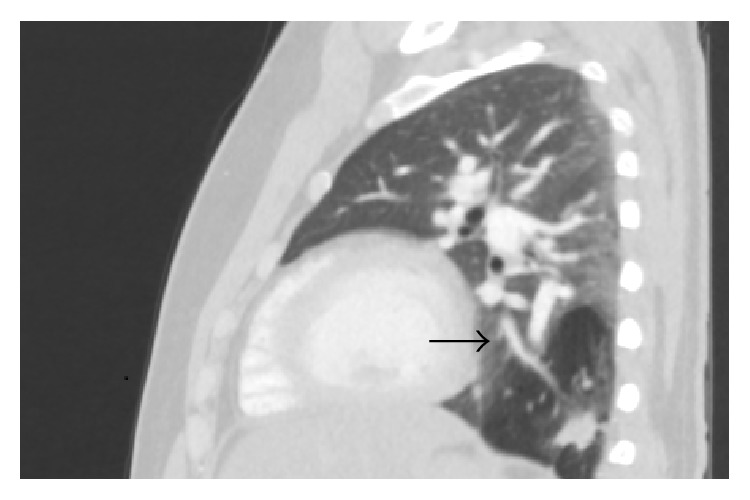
CT thorax sagittal image showing hypodense lesion in the left lower lobe posteriorly with resolving infiltrates within. Arrow: pulmonary vein branch.
